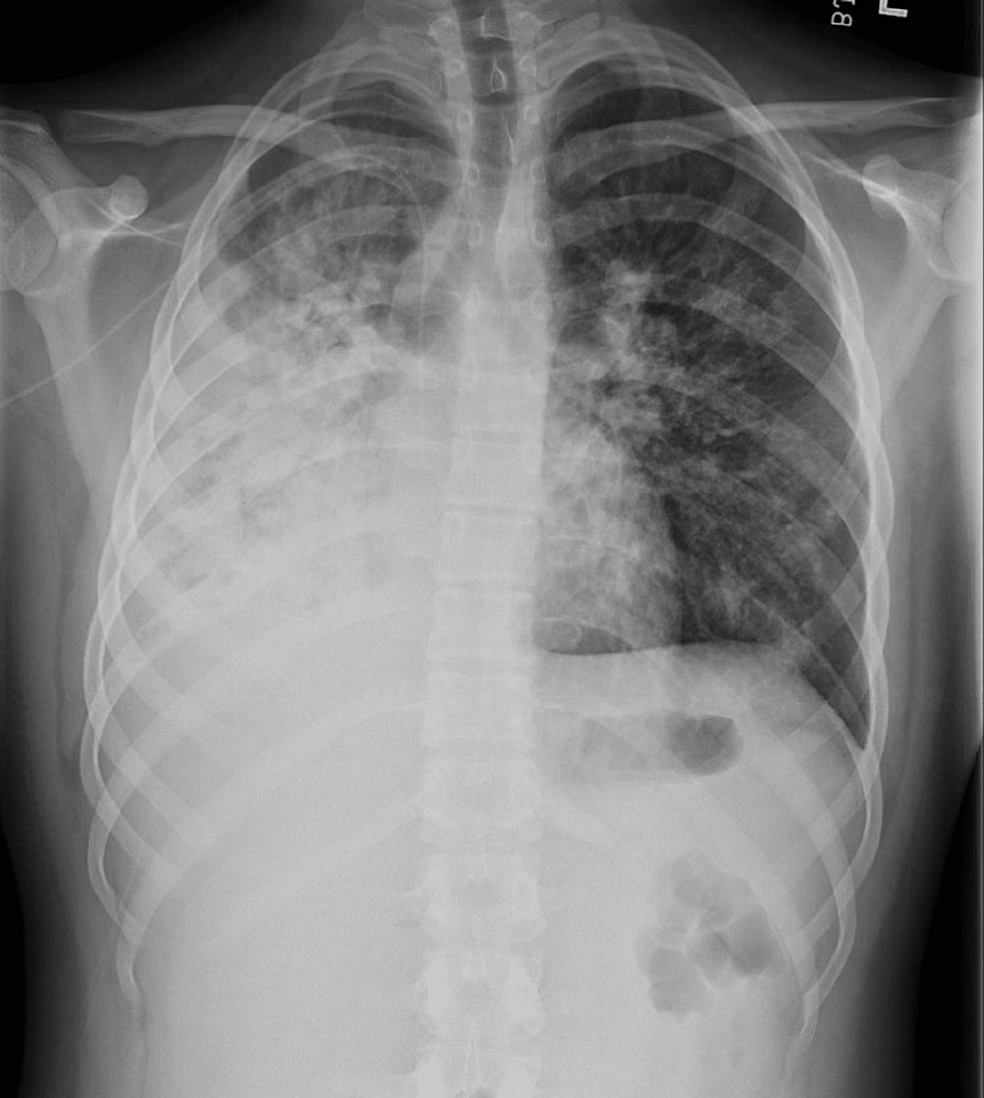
Chest X-rays after two weeks of treatment Extensive airspace disease was seen within the right mid and lower lung zone. There was minimally improved aeration in the right lower lobe. Right pleural effusion was identified as well. Patchy airspace disease was seen throughout the left mid and lower lung zone as well.
